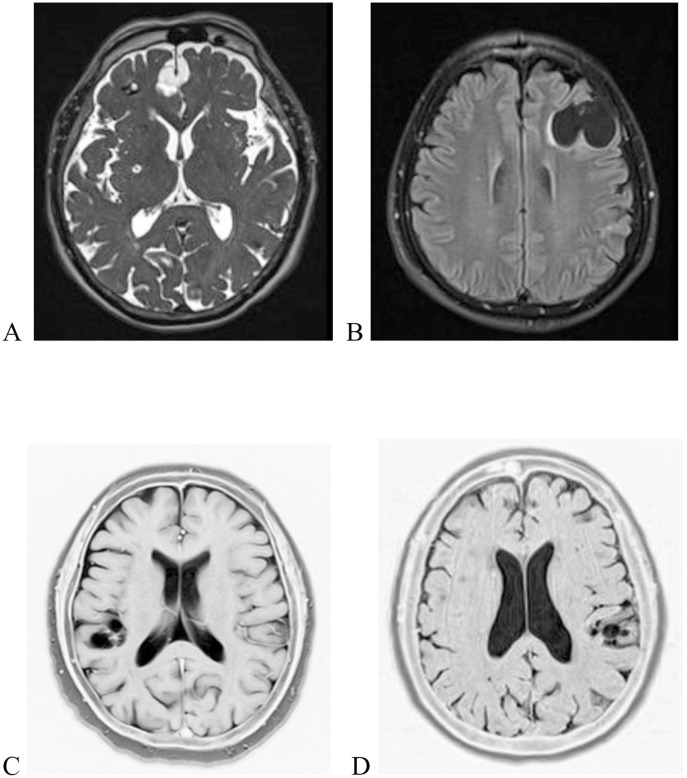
Representative images of brain MRI findings in subjects with radiologically confirmed SANCC. A. Multiple intra-axial and extra-axial viable cysticerci in vesicular stage (subject 2), T2-weighted turbo spin-echo sequence; B. Viable cysticerci occupying the left frontal region with an extra-axial location (subject 1), T2-weighted STIR dark-fluid sequence; C. Viable cysticerci occupying the right parietotemporal lobe, with an extra-axial location (subject 4), T2-wighted turbo spin-echo sequence (grayscale inverted); D. Viable cysticerci clusterized in the parietal left parietal sulcus (subject 3), T2-weighted SPACE sequence (grayscale inverted).
